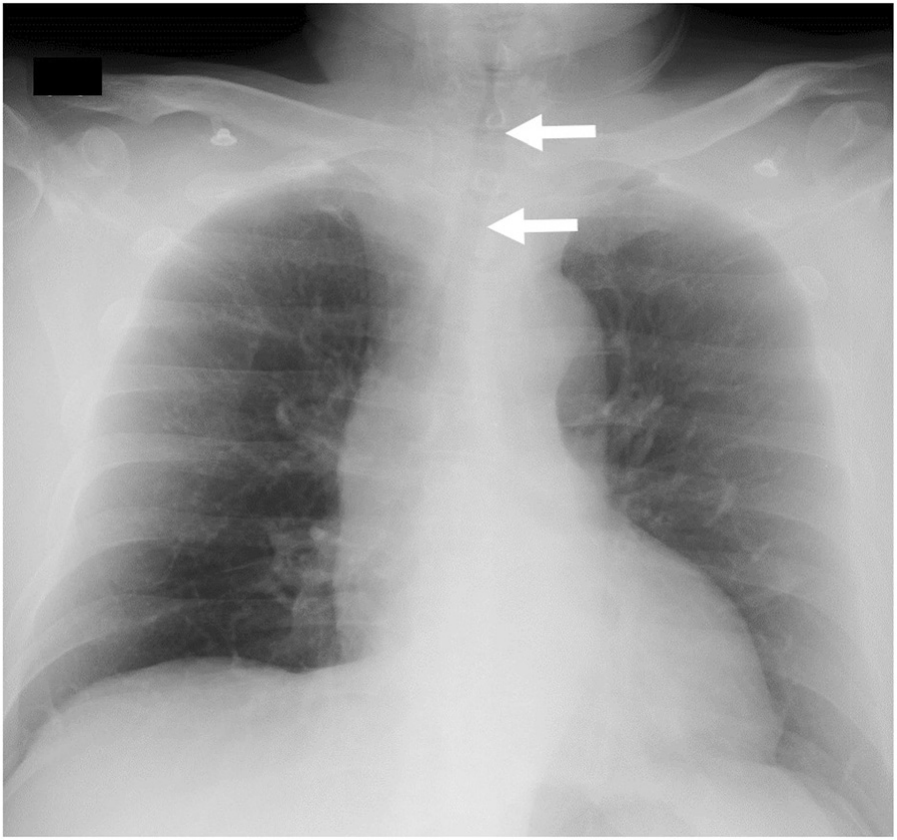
A chest X-ray taken on admission. The chest X-ray image indicated narrowing of the trachea (arrows).
